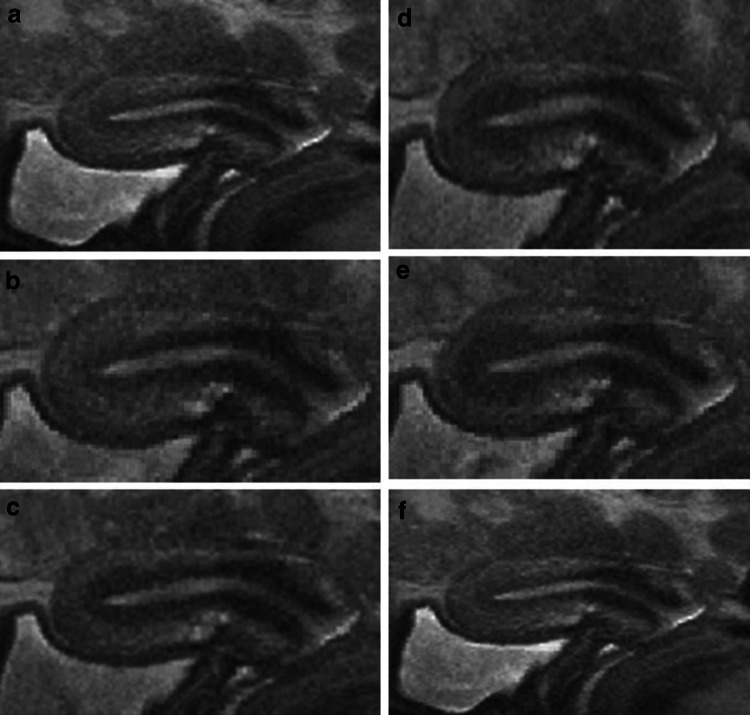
The course of a peristaltic wave of the archimyometrium as shown by a sequence of MRI scans obtained from cinematographic MRI scan in a healthy woman in the late follicular phase. Initially, the archimyometrium appears to be relaxed, indicated by a thin JZ with a less marked hypointensity (a). The peristaltic wave starts with tension of the archimyometrium in the lower half of the uterine corpus, indicated by marked hypointensity of the JZ (b). The zone of increased tension (marked hypointensity) moves in a fundal direction. A muscular package is built up, indicated by the rapid increase of the JZ as the wave moves in a fundal direction (c–e) followed by a rapid relaxation (f)
